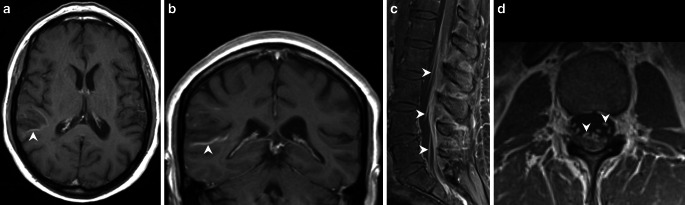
On axial (a) and coronal (b) T1-weighted images after administration of gadolinium the lesion located in the right temporal lobe shows slight rim enhancement (a, b, *arrowheads*). On sagittal (c) and axial (d) T1-weighted postcontrast images of the lumbar spine marked contrast enhancement of the spinal nerve roots in the cauda equina is visible (c, d, *arrowheads*). On-going chemoradiotherapy. The hyperintense signal intensity changes have markedly increased on axial T2-weighted images (a, *arrowhead*)
